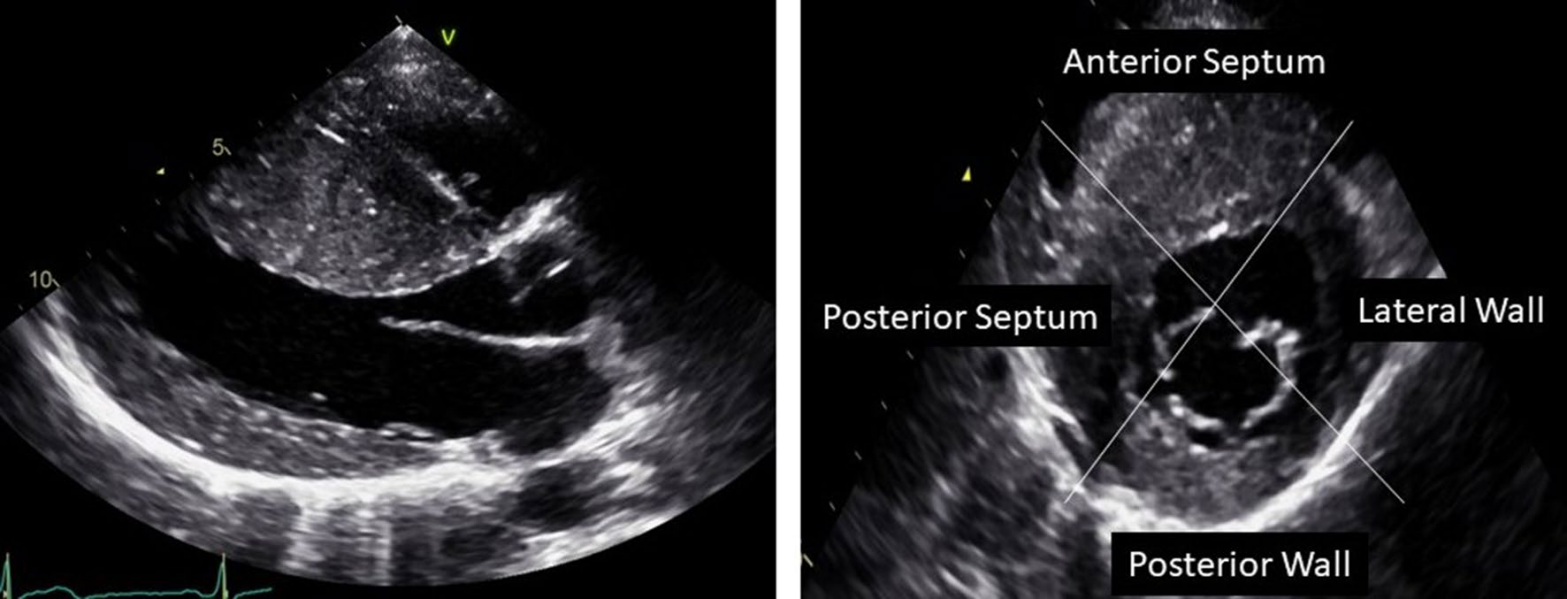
Parasternal long axis and short axis view of an HCM patient with distribution of hypertrophy especially in the septum with MWT of 30 mm
